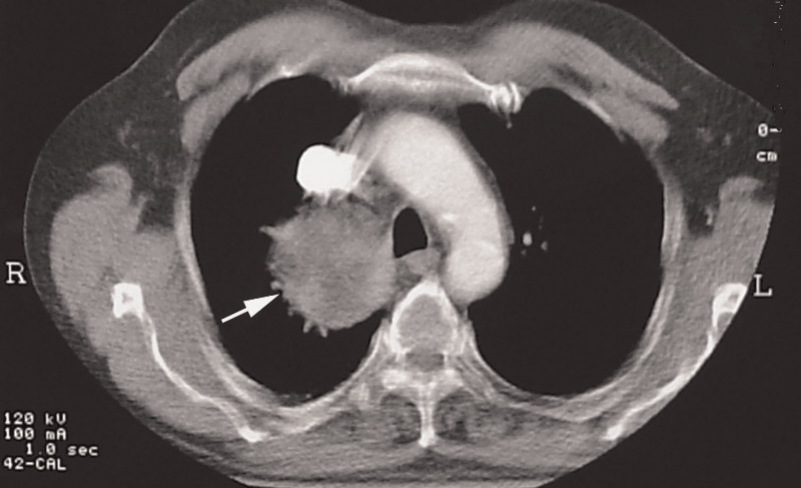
Contrast-enhanced CT demonstrates an inhomogeneous enhancing mass at the right side of the mediastinum (arrow).
